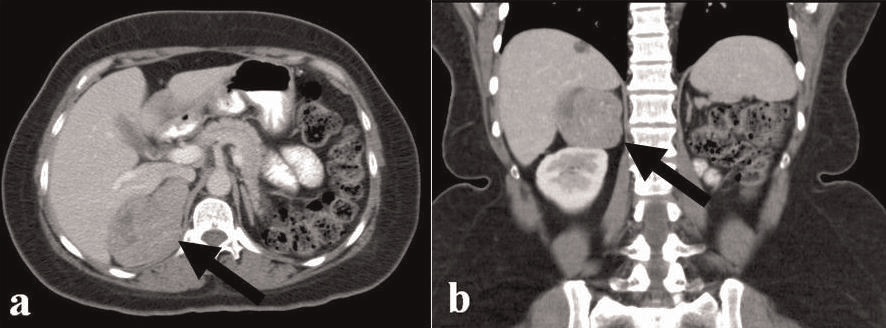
Abdominal CT scan showing a large right adrenal mass.(max diam 6.5 cm).
